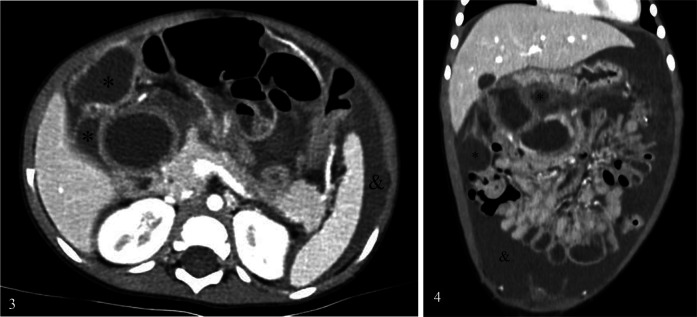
CT scan showing multiple cysts (*) and free fluid (&).
